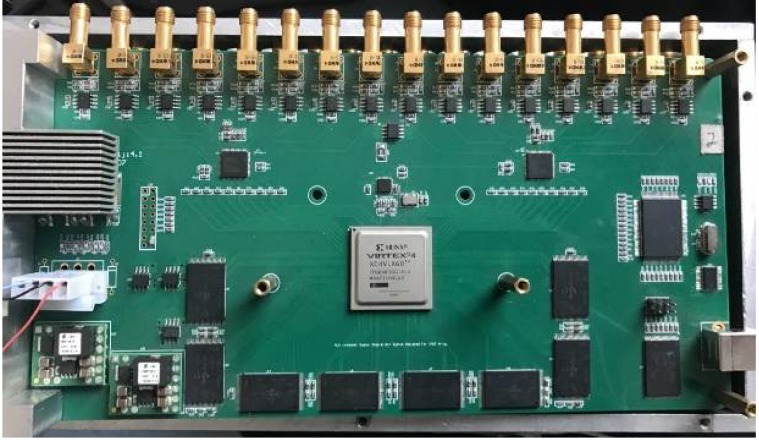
Ultrasonic transmitting/receiving acquisition circuit.
